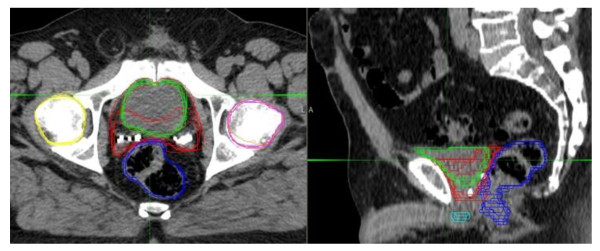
Axial and Sagittal Computed Tomography Image Demonstrating Individual Contours From the Expert Panel. Colors: red represents the contours for the prostate bed; green represents the contours for the bladder; pink represents the contours for the left femoral head; yellow represents the contours for the right femoral head; royal blue represents the contours for the rectum; and teal represents the contours for the penile bulb.
